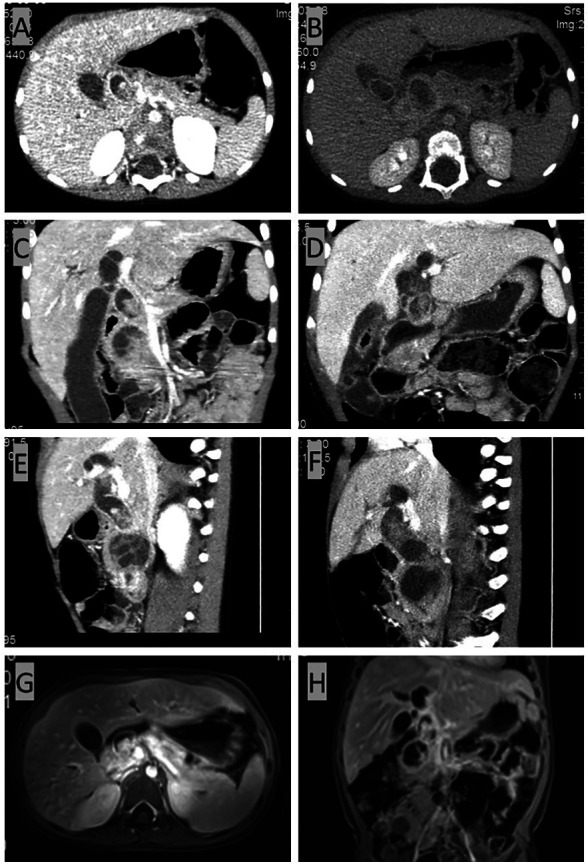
CT and MR results before radical surgery. (A,C,E) CT scan of the abdomen and pelvis with intravenous contrast agent showing an ill-defined 2.9 cm  × 2.8 cm mass with delayed enhancement of the uncinate process with proximal dilatation. (G,H) MR imaging showing a 2.6 cm × 3.6 cm × 2.1 cm mass originating from the common bile duct (CBD), presenting an equal signal on T1WI and an equal-high signal on T2WI. The common bile duct (CBD) was plugged up completely by the mass, resulting in the dilatation of the proximal biliary system. The dilatation of the common bile duct (CBD) reached 2.2 cm in diameter, and the pancreatic duct also presented a slight dilation of 0.1 cm. (B,D,F) CT scan for reevaluation showing the enlarged mass.
